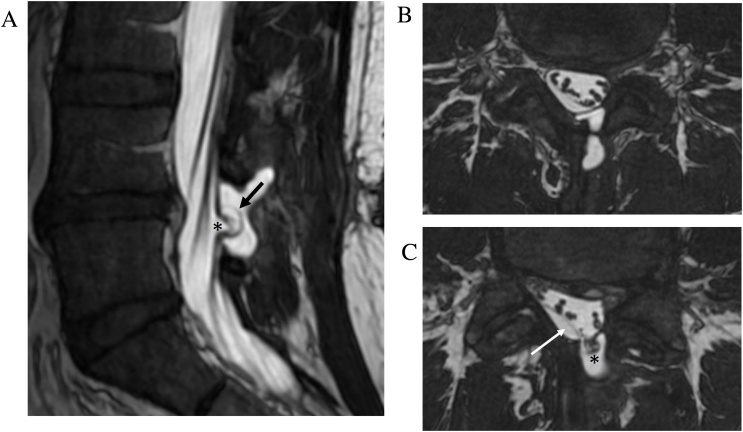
Post-operative pseudomeningocele with nerve root tethering. Sagittal FIESTA-C MRI image (a) shows a dural defect (arrow) and adjacent pseudomeningocele at the site of prior L4-5 laminectomy. Axial FIESTA-C MRI images (b–c) There is tethering and herniation of traversing sacral nerve roots (asterisk) through the dural defect. A subdural effusion with displacement of the arachnoid membrane (arrow) is also present.
